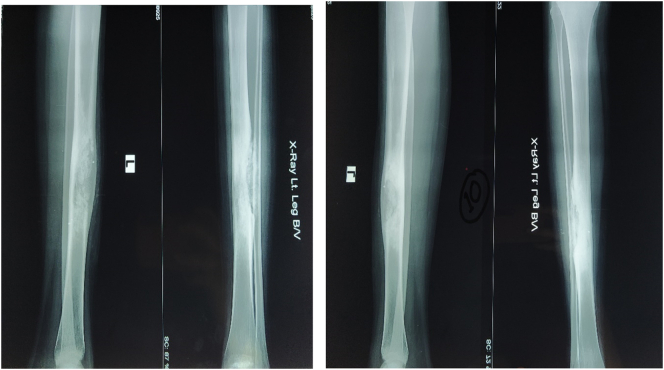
Follow-up X-ray taken after 6 months and 1 year.
